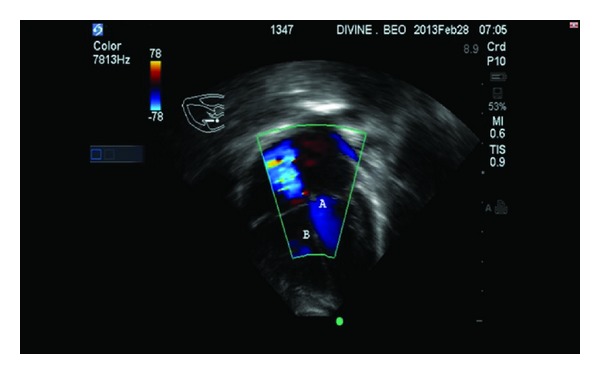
Color flow Doppler showing the tricuspid leaflet with no tricuspid regurgitation. (A) indicates LV. (B) shows the RV.
